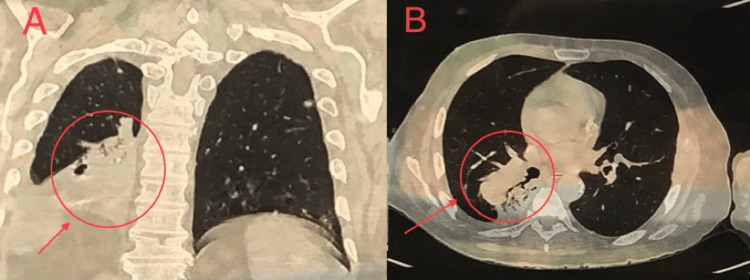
Contrast enhanced CT scan of the chest showing right lower lobe partly spiculated partly lobulated lung lesion in coronal section (A) & transverse section (B).
